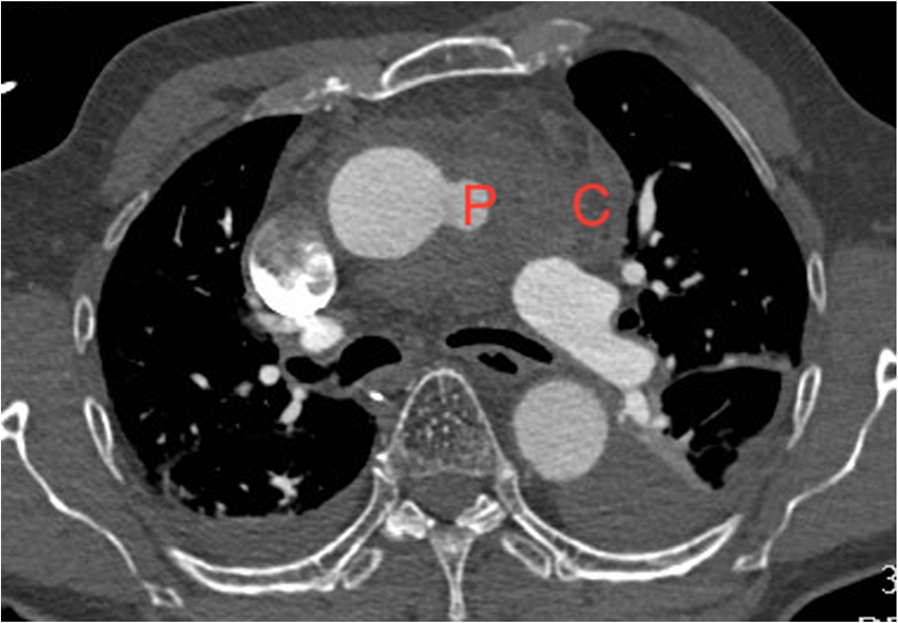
CT scan showing large pseudoaneurysm (P) in relation to the distal ascending aorta with rupture into adjoining soft tissue with considerable walled mediastinal clot (C) and left hemothorax
